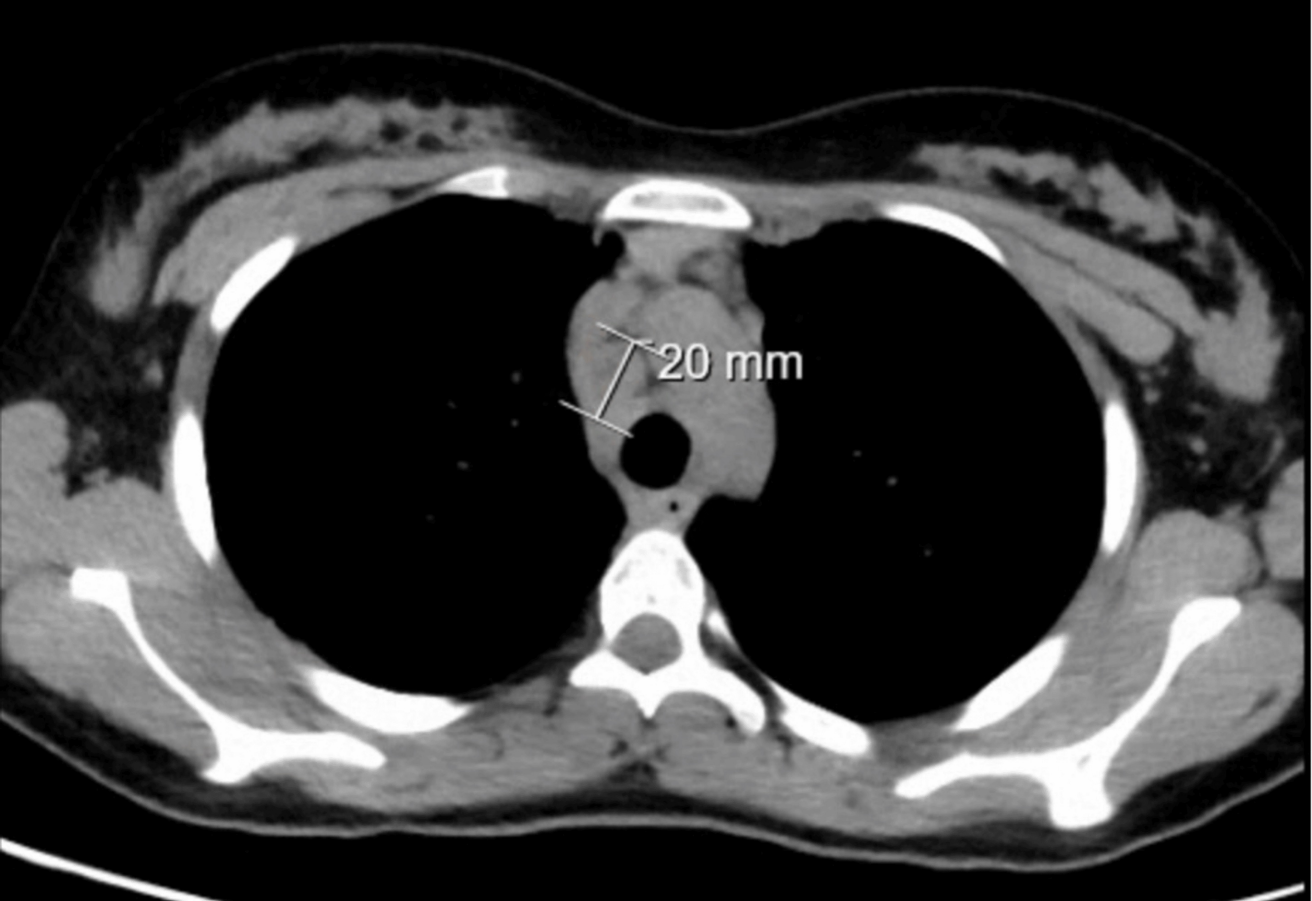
Axial plane of chest computed tomography in mediastinal view Mediastinal lymphadenopathy with nodes measuring up to 20 mm.
